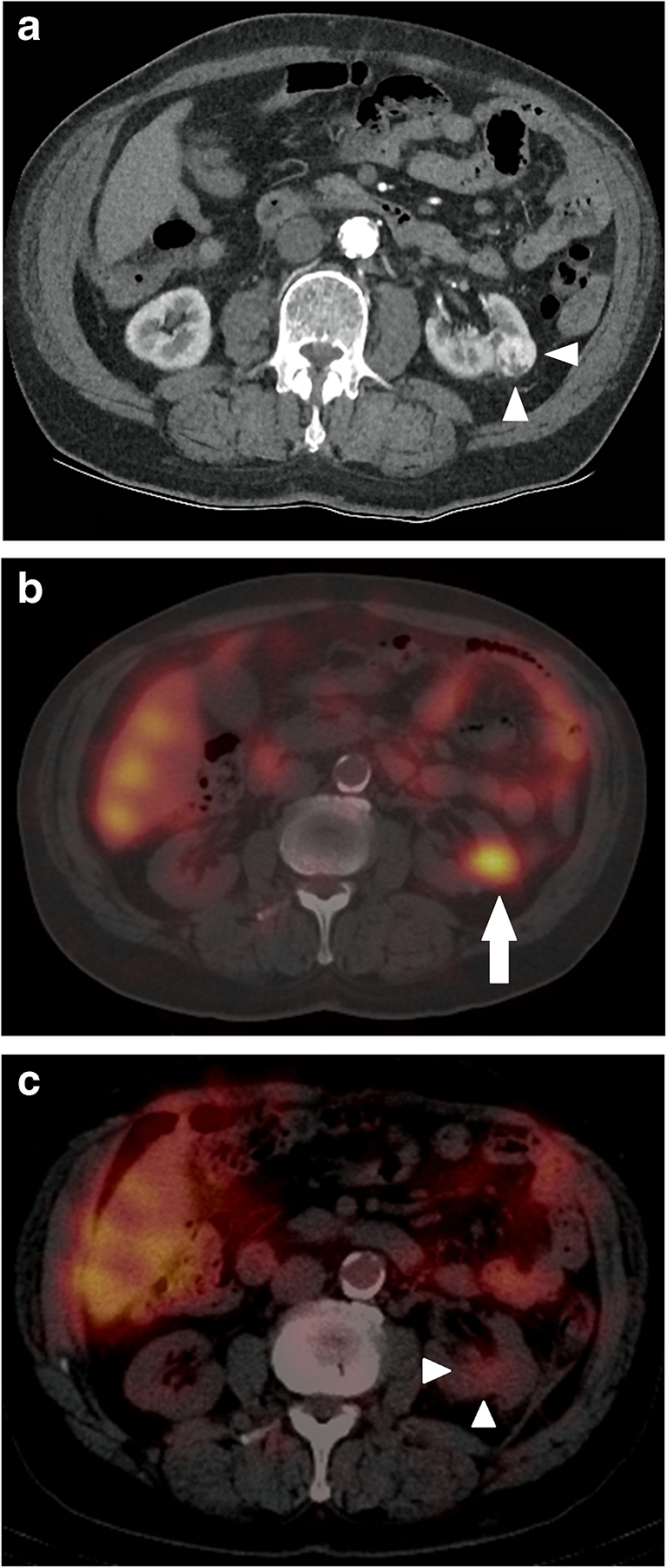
a. In this 70-year-old male patient, an incidentaloma was found in the left kidney on ultrasound. This axial contrast enhanced CT image in the corticomedullary phase confirms the presence of a 25 mm process suspicious for malignancy (white arrowheads). Biopsy confirmed clear cell renal cell carcinoma. b. Axial SPECT image showed uptake of the lesion (white arrow) before cryoablation was performed. c. One month after percutaneous cryoablation SPECT showed no uptake of the ablated lesion. Very low uptake was seen surrounding the ablated lesion (white arrowheads)
